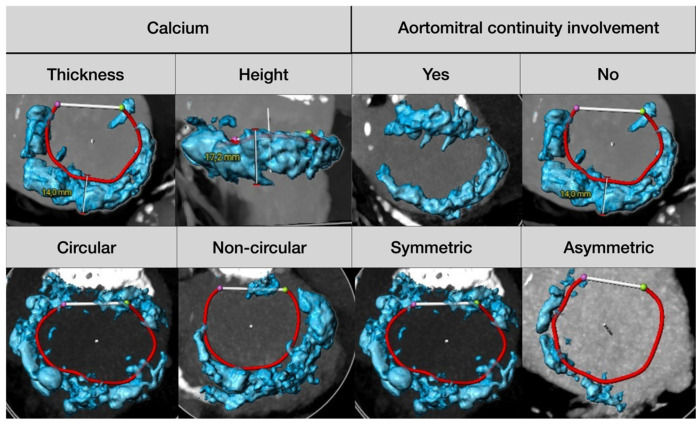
CT-derived MAC parameters. Insights in assessment of quantitative and qualitative MAC patterns. CT—computed tomography, MAC—mitral annular calcification.
